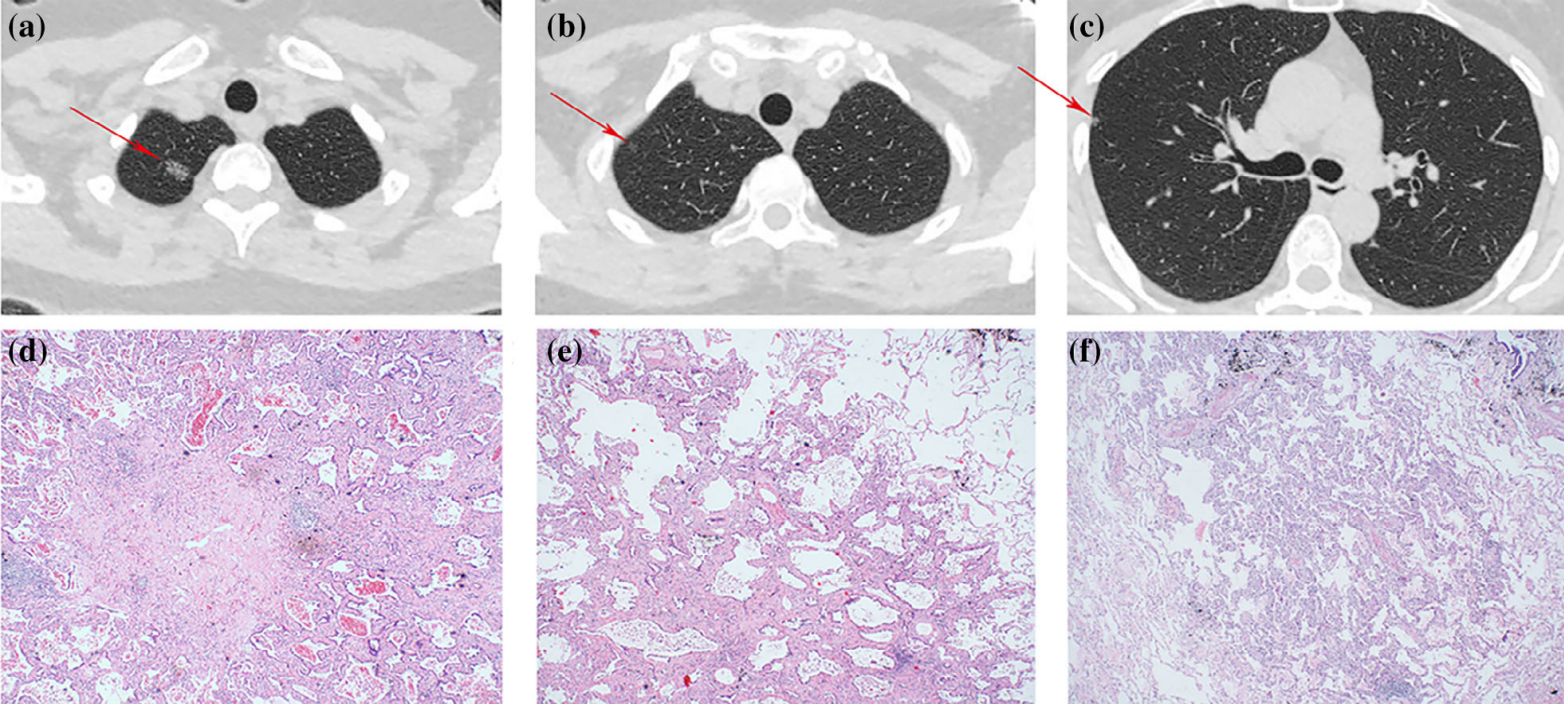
(a, b, c) Lung computed tomography (CT) scan. The patient's preoperative lung CT scan is shown; the red arrow indicates the tumors located in the right upper lobe of the lung (a, b, and c refer to IA, MIA and AAH, respectively). (d, e, f) Pathological images (d, e, and f refer to IA, MIA and AAH, respectively).
